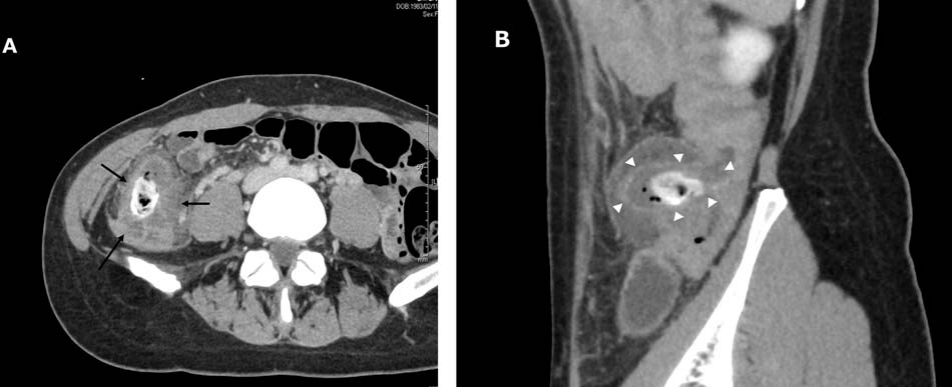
Abdominal and pelvic CT with intravenous contrast at the time of consultation. Axial image (A) demonstrated cecal colon diverticulum (black arrows) and sagittal image (B) demonstrated thickening and mural edema of the diverticulum (white arrowheads), indicating inflammation.
